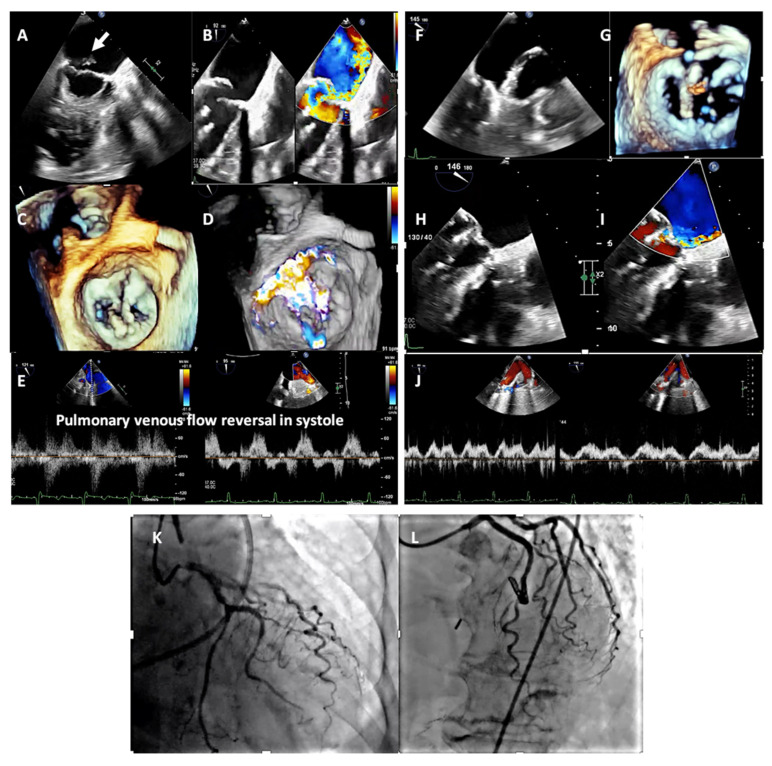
(A) 74-year-old woman who presented with acute onset chest pain and cardiogenic shock, hypoxemic respiratory, and multiorgan failure. She underwent emergent intubation, inotropic support, and an intra-aortic balloon pump. (A,C); papillary muscle rupture (arrow) on transesophageal echocardiogram (TEE). LV end-diastolic volume and EF were 65 mL/m^2^ and EF 65%, respectively. (B,D,E); findings of severe eccentric mitral regurgitation by 2D and 3D color Doppler and pulsed wave Doppler interrogation of pulmonary venous flow with systolic reversal, (F,G); TEE guided emergent TEER using 1 MitraClip XTW device, (H,I); immediate post M-TEER result with systolic blood pressure increasing to 130 from 90 mmHg and a decrease in left atrial pressure from 30 mm Hg to 15 mmHg, (J); restoration of normal pulmonary venous flow pattern, (K); Coronary angiography showing lesions in the left anterior descending and circumflex arteries in the acute phase, (L); result of coronary stenting 2 weeks after the hemodynamic stabilization with medical treatment and M-TEER.
